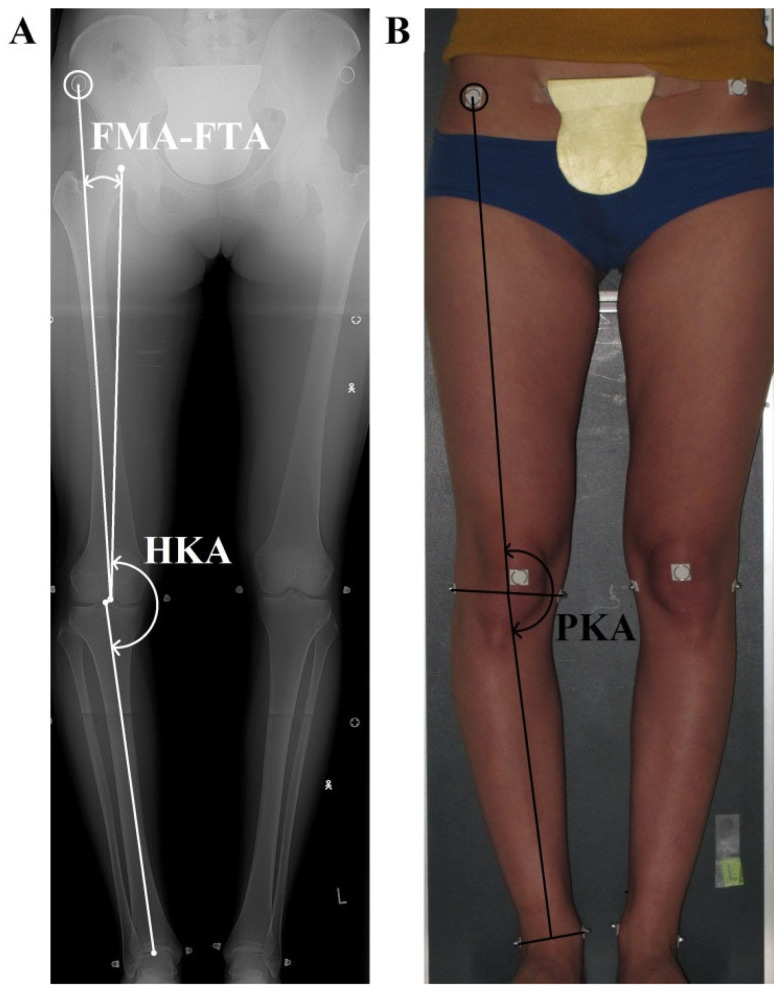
The measured angles. Both pictures were taken of the same patient at the same time, one after the other. The patient stood in the standardized position. (A) Radiograph: hip–knee–ankle angle (the HKA, formed by femoral and tibial mechanical axes, with values < 180° indicating varus); the FMA-FTA angle formed by the femoral mechanical axis and the radiographic femoral topographic axis; (B) photograph: pelvis–knee–ankle angle (PKA, formed by the photographic femoral and tibial topographic axes).
